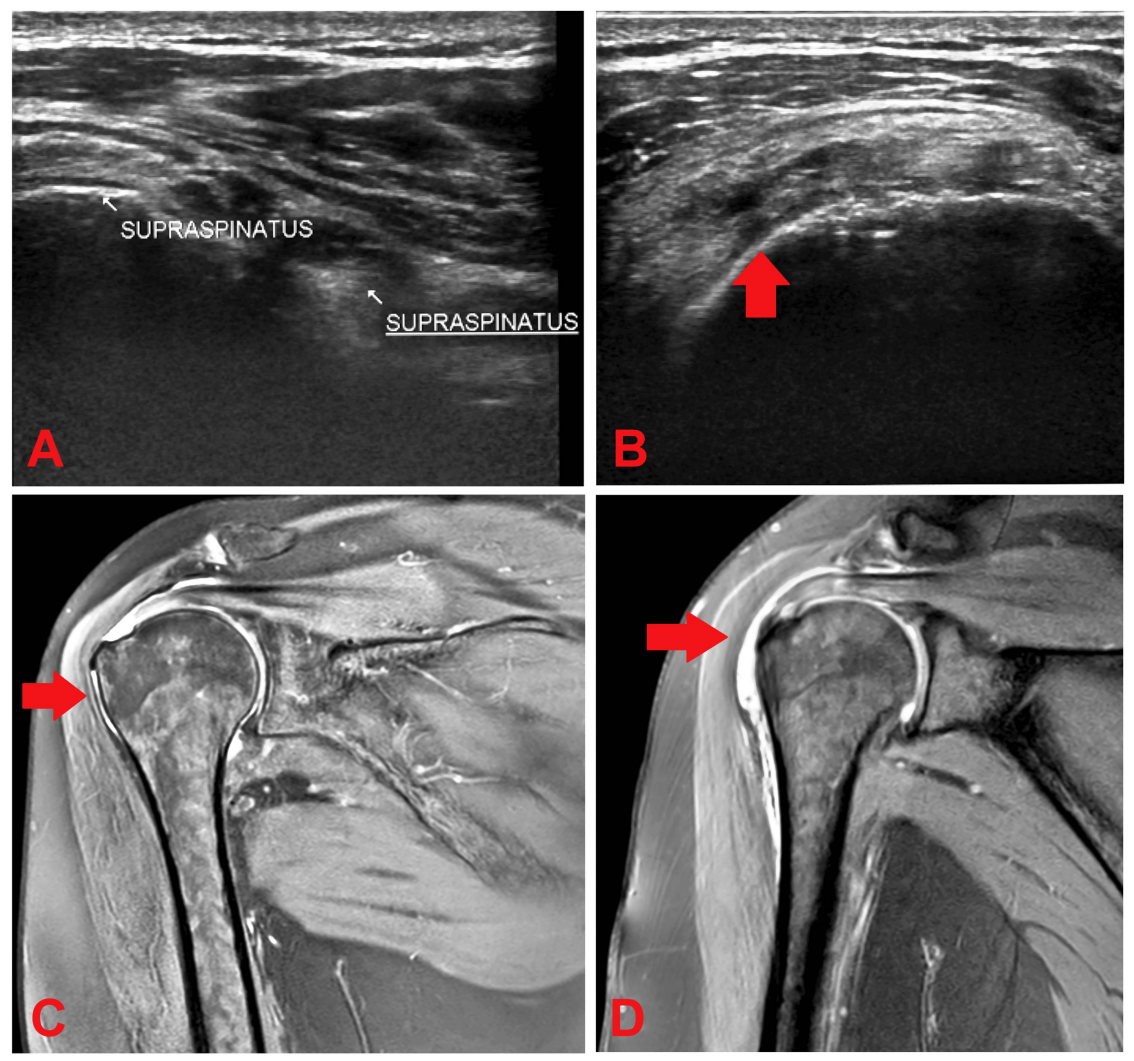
(A) USG image of supraspinatus complete tear; (B) USG image of supraspinatus partial tear; (C) MRI image of supraspinatus complete tear; (D) MRI image of supraspinatus partial tear Image credit: Dr. Bhagyasri Nunna USG: high-resolution ultrasound; MRI: magnetic resonance imaging
